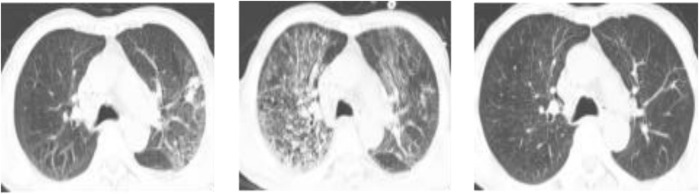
Images of chest CT scans acquired overtime showing changes associated with therapeutic interventions. (A): on arrival, chest CT showed infectious lesions in the left lung; (B): before the treatment, bilateral lung lesions progressed; (C): after treatment, significant improvement was revealed.
